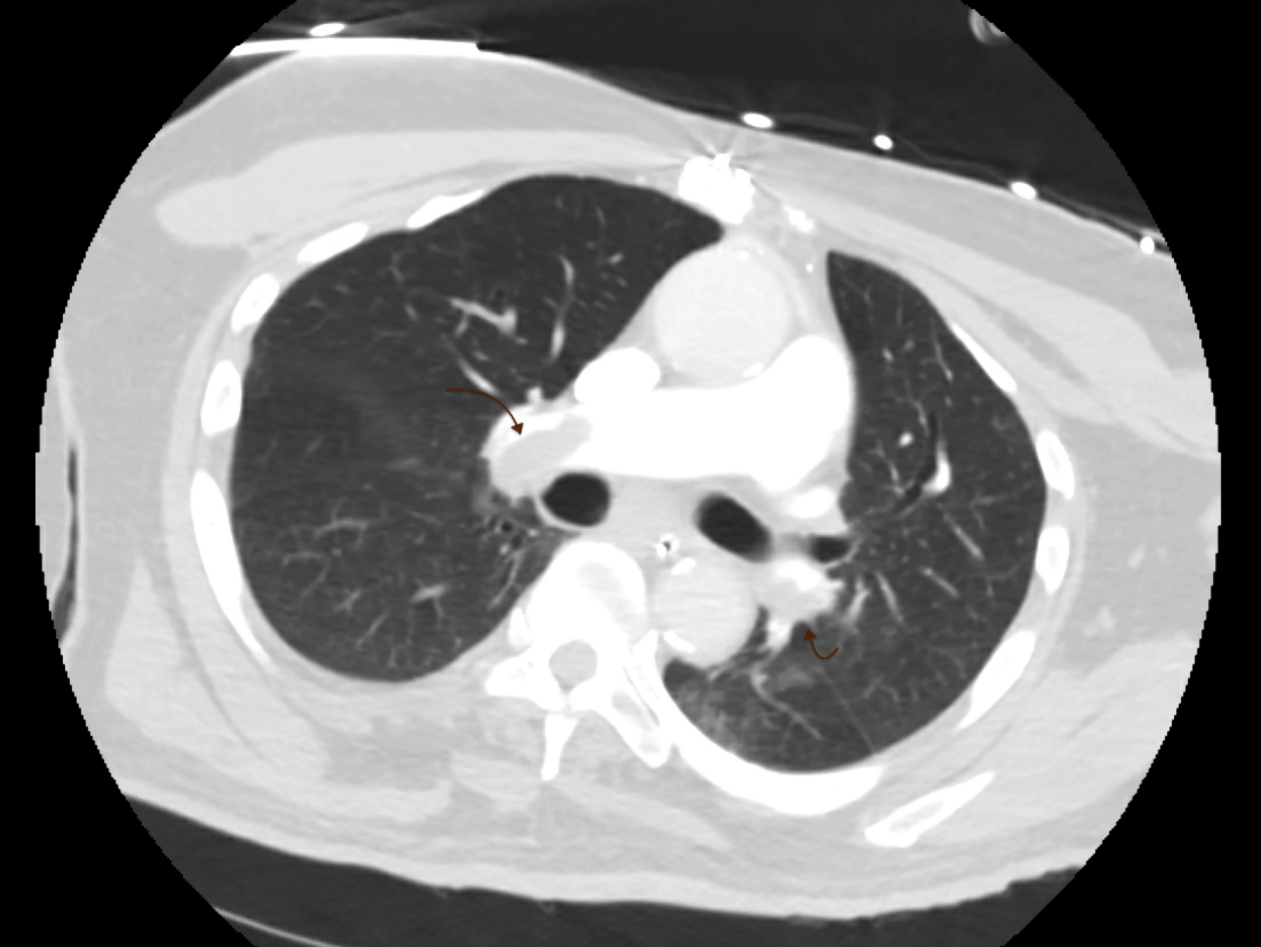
CT With Contrast The presence of extensive bilateral central pulmonary emboli with filing defects.
